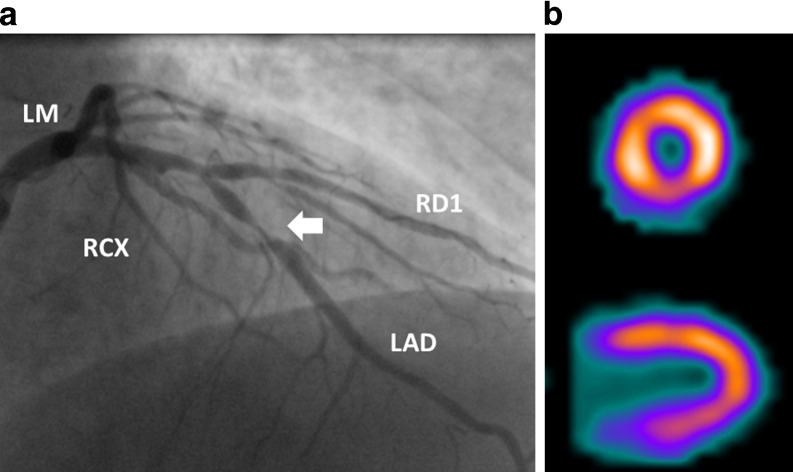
Coronary angiogram showing significant stenosis (arrow) in mid-segment of LAD (a) in a patient with persisting angina and normal SPECT MPI (b). (*LAD* left anterior descending artery, *LM* left main coronary artery, *RCX* right circumflex artery, *RD1* diagonal branch)
